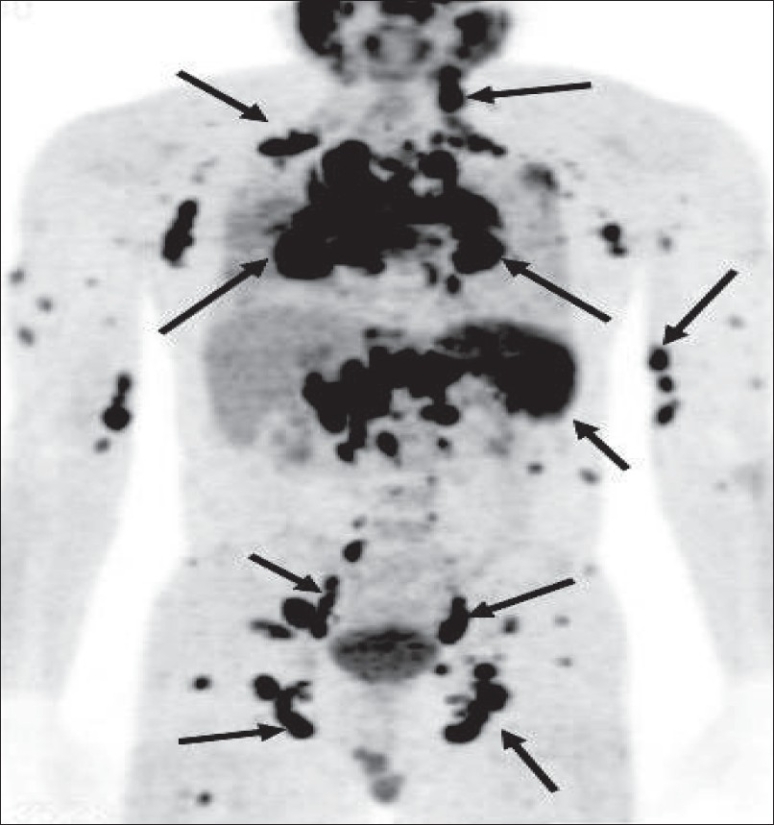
MIP image from a PET study of a 42-year-old man with widespread intensely FDG-avid lesions involving lymph nodes in the neck, supraclavicular regions, axillae, chest (bilateral hilar and mediastinum), abdomen (para-aortic, retroperitoneal and iliac chain) and inguino-femoral regions as well as the spleen along with multiple subdermal/ subcutaneous lesions (arrows), mimicking lymphoma. Biopsy showed sarcoidosis in the nodes, as well as in the subdermal/ subcutaneous lesions
